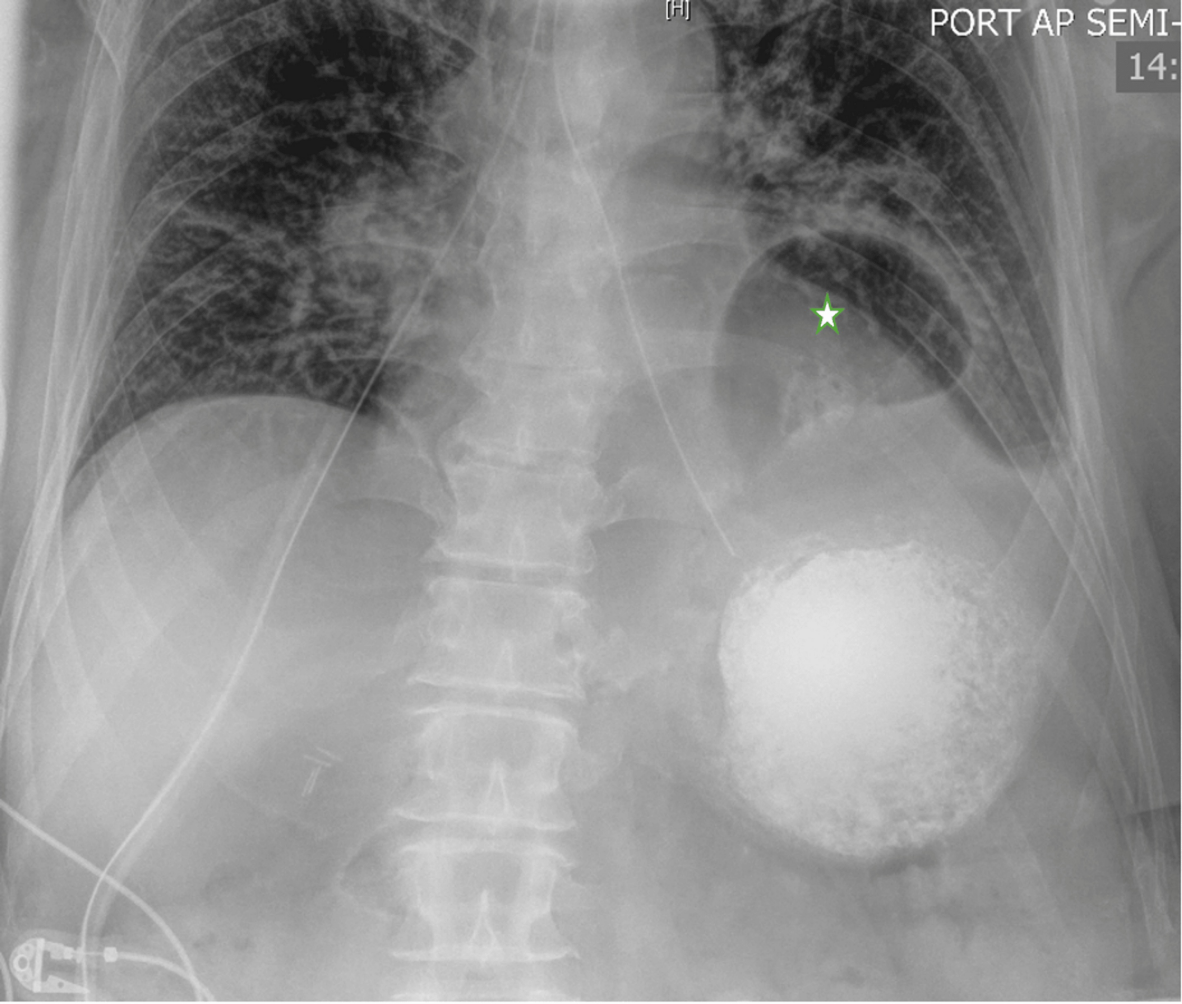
Abdominal X-ray image The image displays a hiatal hernia with a substantial portion of the stomach positioned above the left hemidiaphragm (marked with a star).
